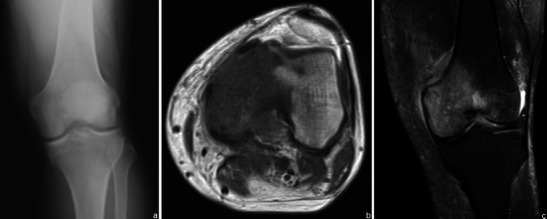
Patient with chronic left knee pain. Radiographs were obtained (a normal frontal radiograph). Subsequent MRI study showed abnormal signal intensity in the bone: a low T1WI signal (b) and T2FS WI hyperintense lesion (c) related to a bone lymphoma
